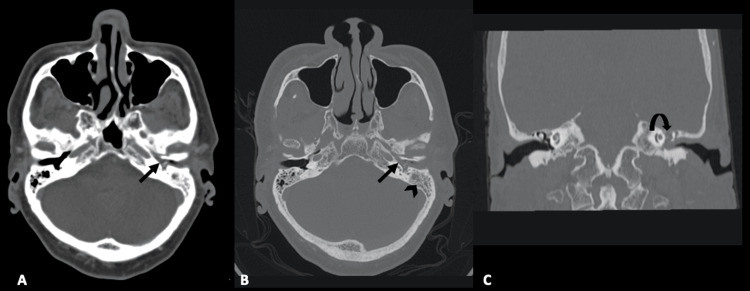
HRCT temporal in (A) axial soft tissue window, (B) axial bone window, and (C) coronal bone window Presence of fluid density within the external and middle ear cavities (long arrow) as well as mastoid air cells (arrowhead) indicating left otitis externa, left otitis media, and left mastoiditis. The normal left tympanic membrane is not visualized. There is a dehiscence of the left tegmen tympani (curved arrow) seen in image (C), suggestive of bony erosion. HRCT: high-resolution computed tomography
